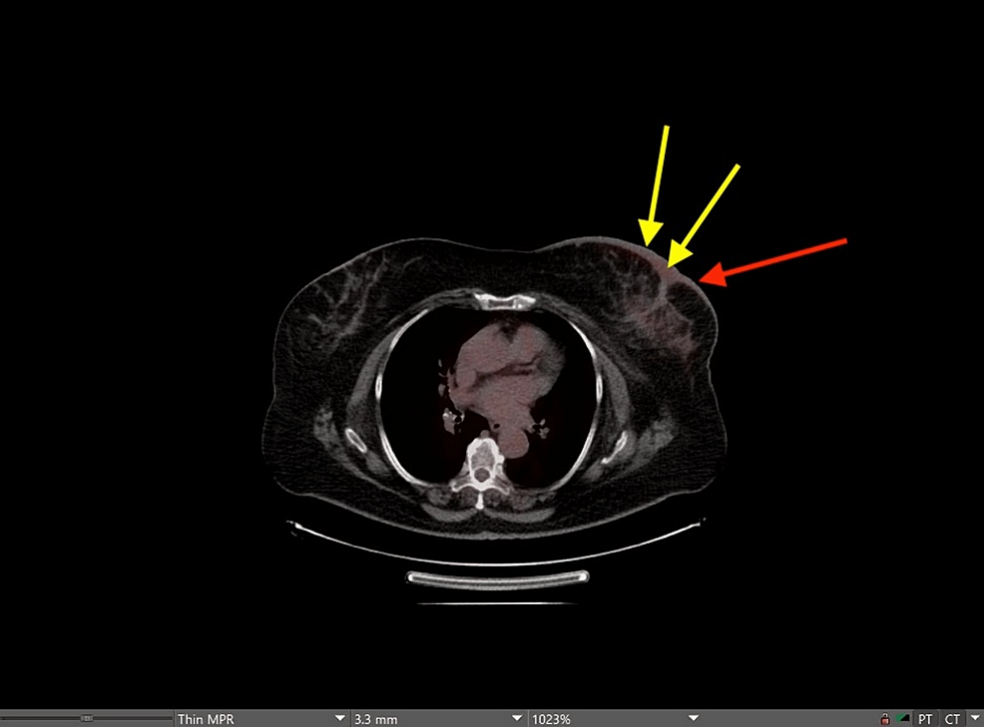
PET scan. Axial PET/CT of the left breast demonstrating increased uptake and skin thickening based on the radiologic impression. The red arrow points to the site of the lesion. Yellow arrows point to the site of increased uptake as demonstrated by the red hue on the image. PET, positron emission tomography
